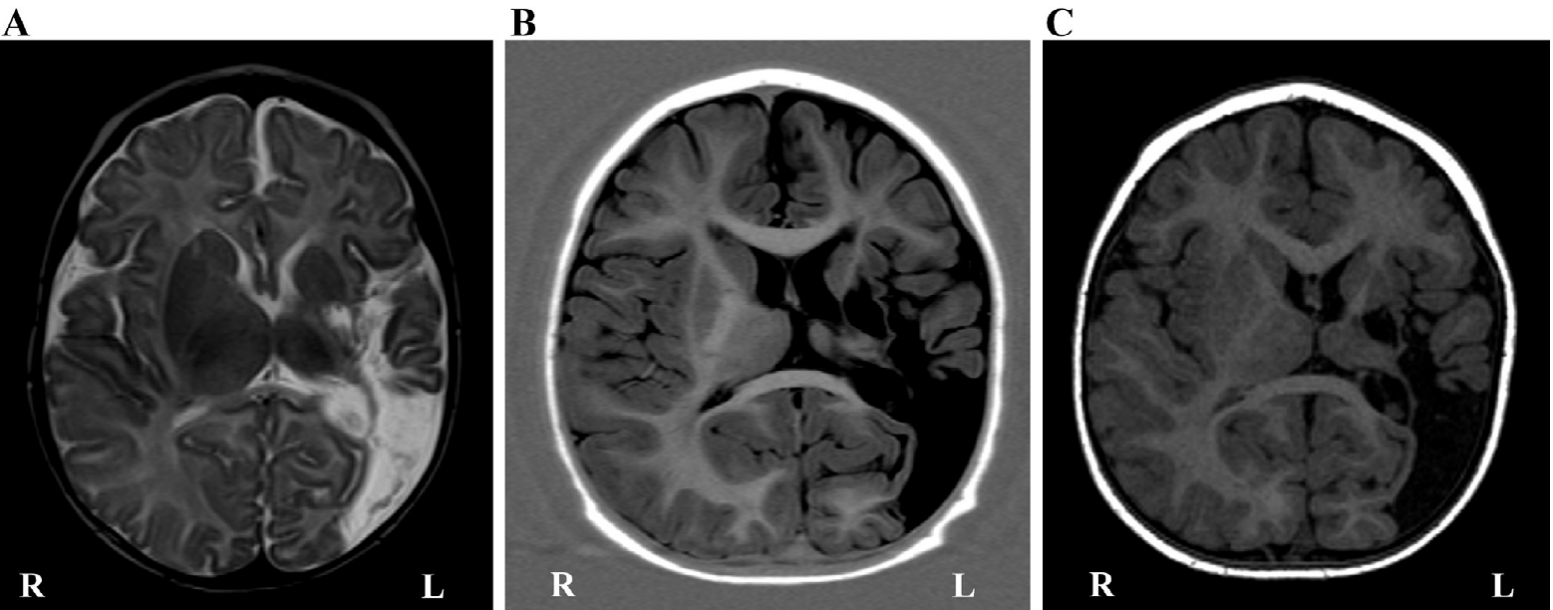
Routine MRI showing the extent of the lesion in the left hemisphere on an axial view, at (A) 3 months of age with T2-weighted image, (B) 12 months of age with an inversion recovery T1-weighted image, and (C) 20 months of age with a T1-weighted gradient-echo image.
